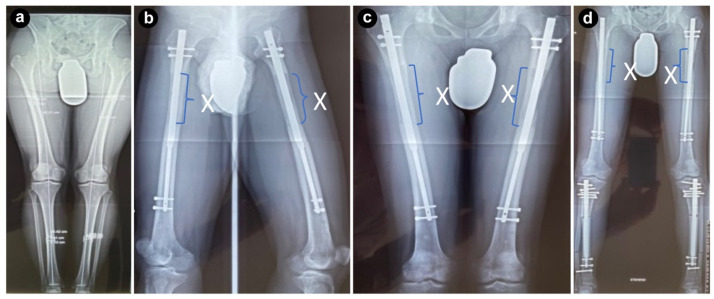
X-rays during each surgical step. X indicating the lengthening area: (a) X-ray prior to the initial surgery, (b) during upper leg lengthening, (c) finished upper leg lengthening, and (d) after removal of irritating proximal screws right upper leg and beginning of lower leg lengthening.
